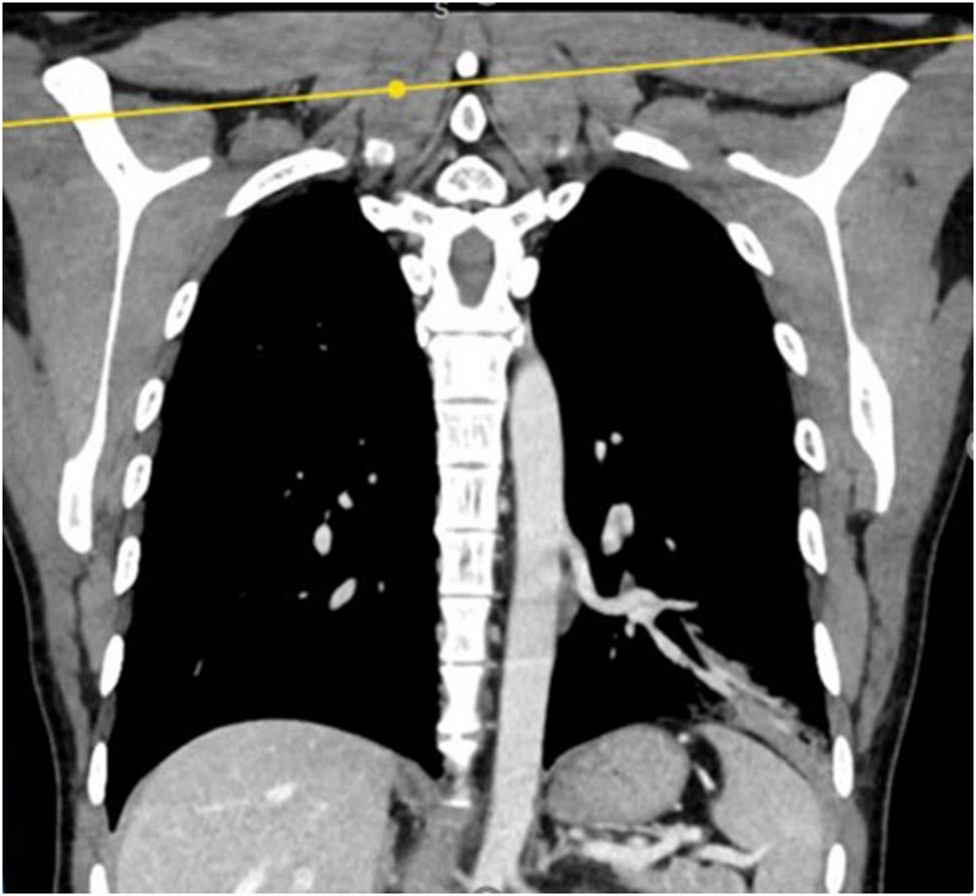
Computed tomography pulmonary angiography (coronal section) shows area of consolidation in left lower lung lobe with feeding vessel originating from descending thoracic aorta.
